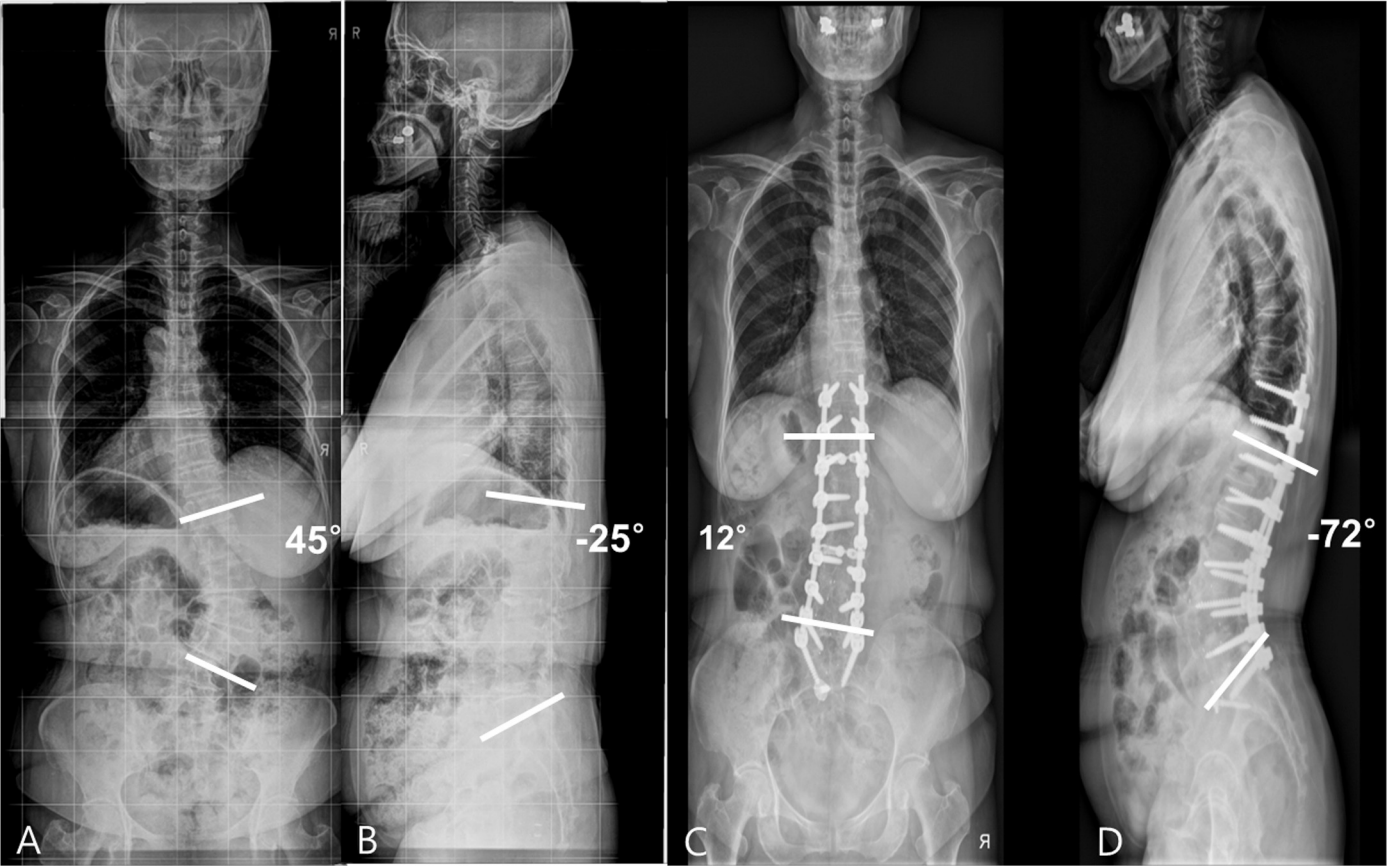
(A, B) Anteroposterior (AP) and lateral standing radiographs of a 65–year–old woman with severe back pain and pain in both legs, showing degenerative lumbar kyphoscoliosis. A three–step (posterior–anterior–posterior) approach was used to obtain adequate sagittal and coronal balance. (C, D) Postoperative AP and lateral radiographs showed L3–S1 interbody fusion and good sagittal alignment.
